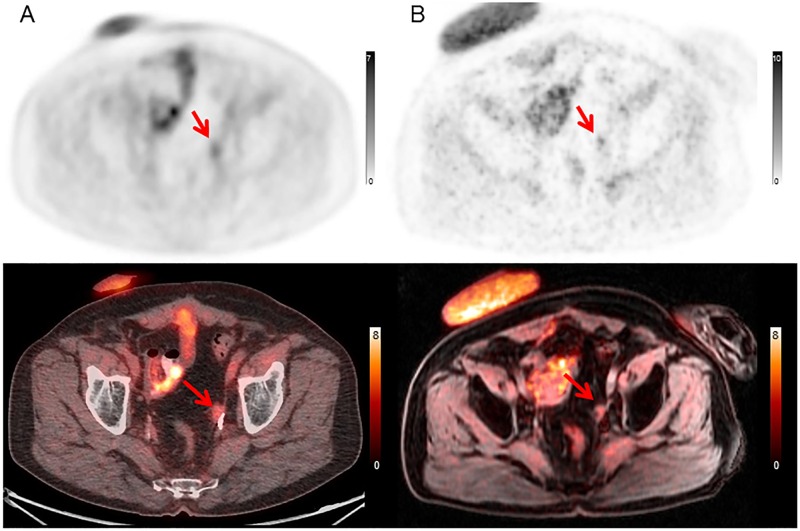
Lesion identified prospectively on PET/CT but only retrospectively on PET/MRI. 65 year-old male with bladder cancer who underwent PET/CT for subsequent treatment strategy. (A) PET/CT image (upper: PET image from PET/CT, lower: fused PET and CT) (B) PET/MRI image (upper: PET image from PET/MRI, lower: fused PET and MRI). PET/CT could identify the small nodular lesion at the left pelvic wall, due primarily to the better spatial localization possible with CT in this case. It was identified on PET/MRI only retrospectively, as on the initial read, it was thought to be activity in adjacent bowel.
